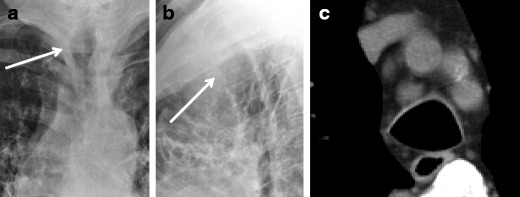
Fifty-nine-year-old man with a frontal, b lateral radiographs, and c axial CT demonstrating a dilated trachea (*arrows*) measuring greater than 3 cm in diameter. These findings are compatible with tracheobronchomegaly
